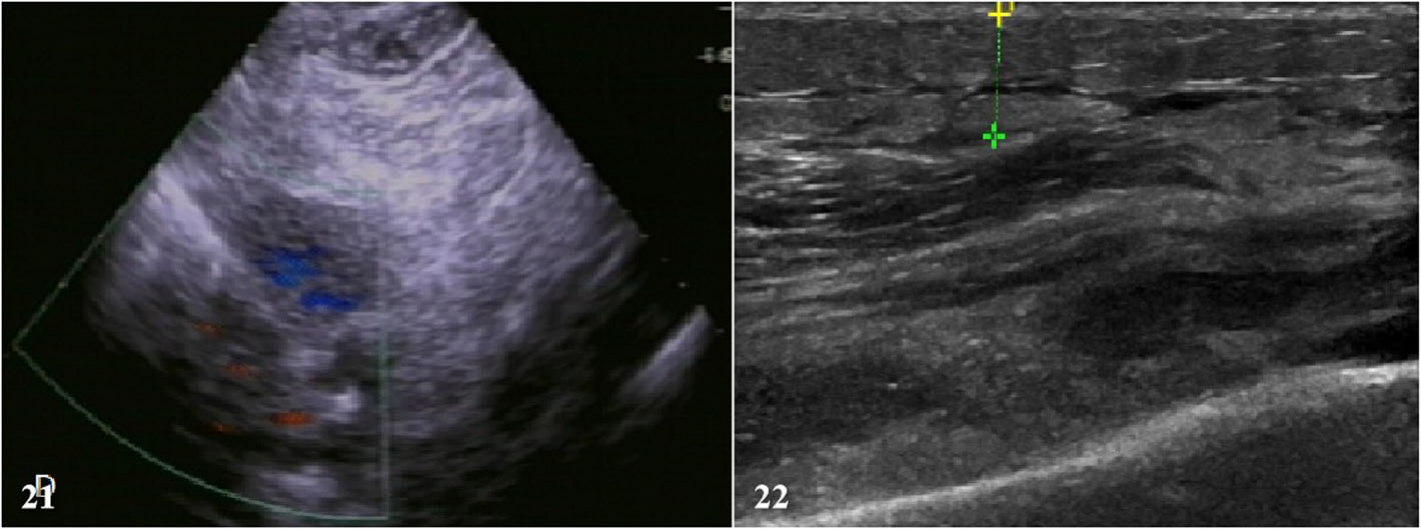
Ultrasound scan on the right knee joint which was suspected to PJI
